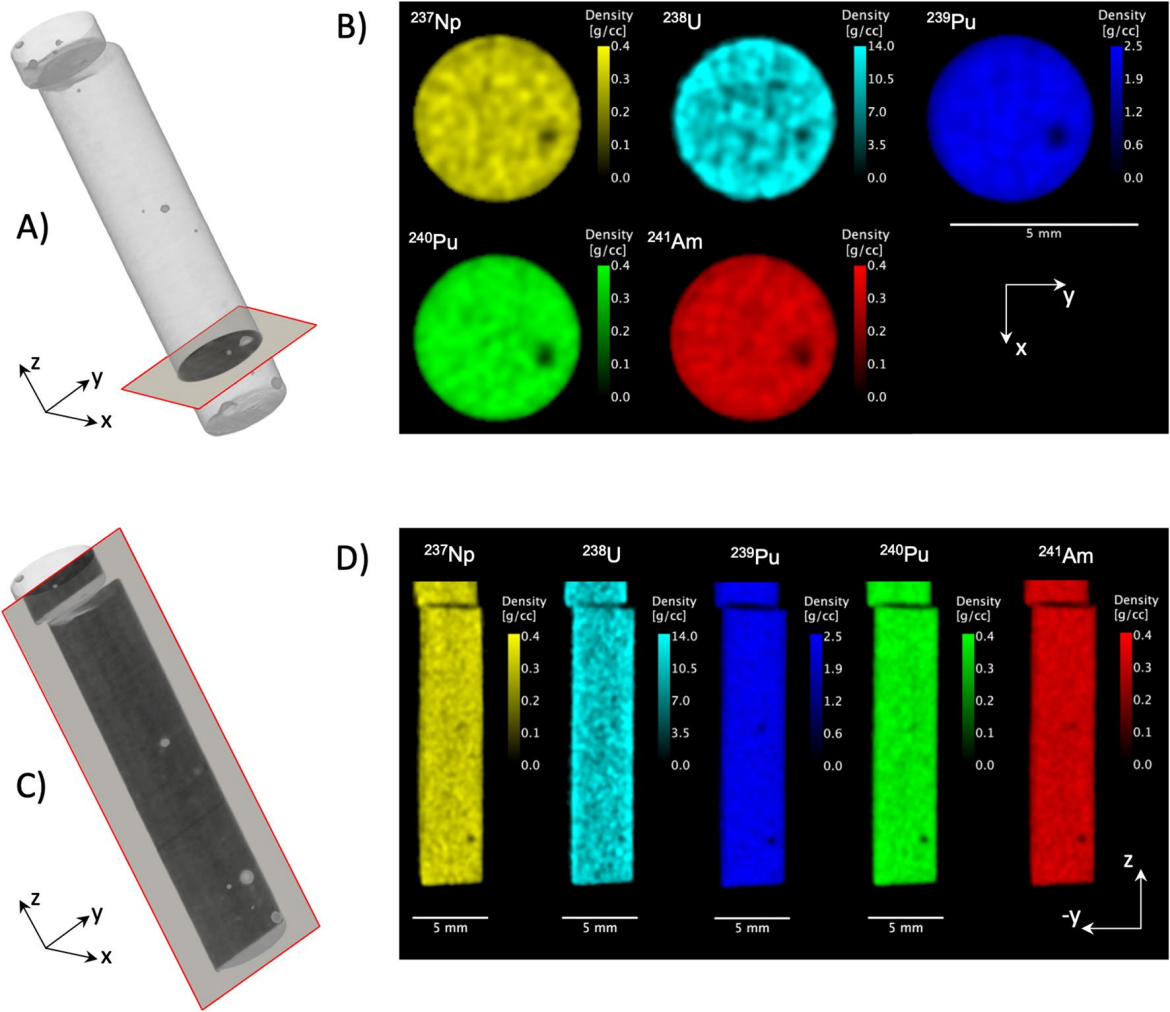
Volumetric reconstruction using epithermal neutrons of the U-20Pu-10Zr-3Np-2Am sample with indicated region in red for CT slices of the volumetric densities of individual isotopes. Slices normal to the cylinder axis in (A) with corresponding isotope densities shown in (B) and slices parallel to the cylinder axis in (C) with corresponding isotope densities shown in (D), respectively.
